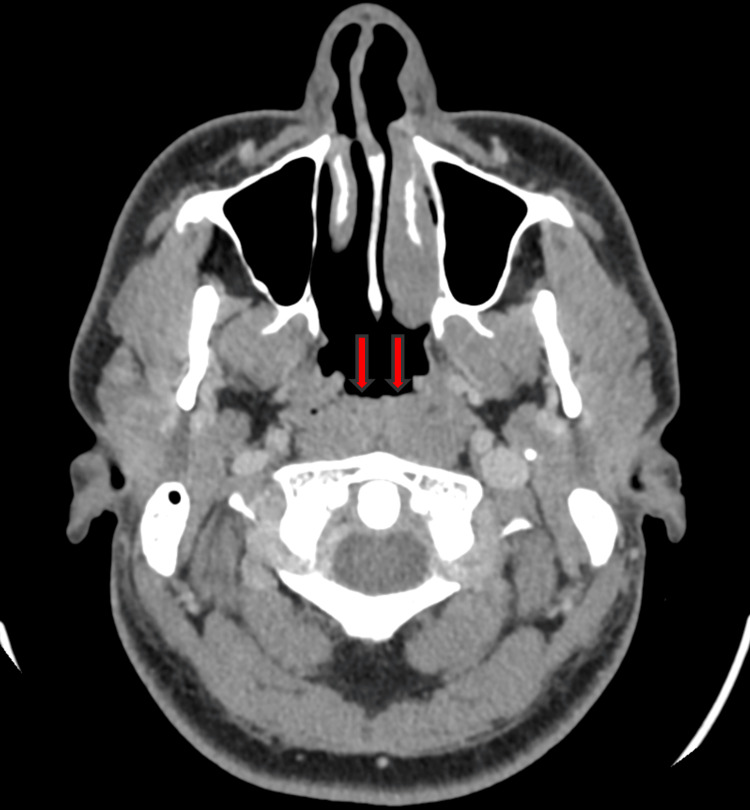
Axial CT image showing homogeneous thickening of the posterior-superior nasopharyngeal walls (arrows)
